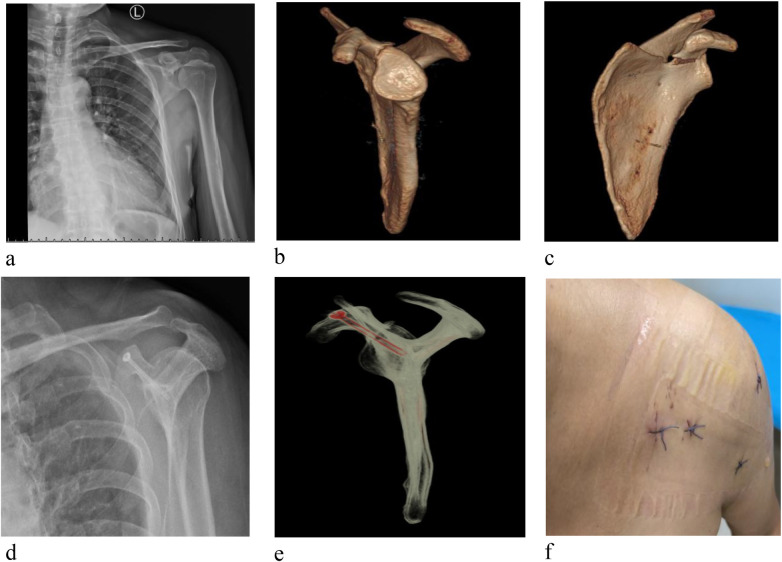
A 51-year-old female was admitted with left shoulder pain following a fall. (a) Preoperative image showing acromioclavicular joint dislocation (Rockwood type II). (b,c) Coracoid process fracture (Ogawa type I, Eyres type IV A). (d) Postoperative Y-view radiograph of the shoulder demonstrating reduction of the acromioclavicular joint. (e) Adequate fixation of the coracoid process fracture with one 3.5 mm screw. (f) Postoperative photograph of the surgical incision.
